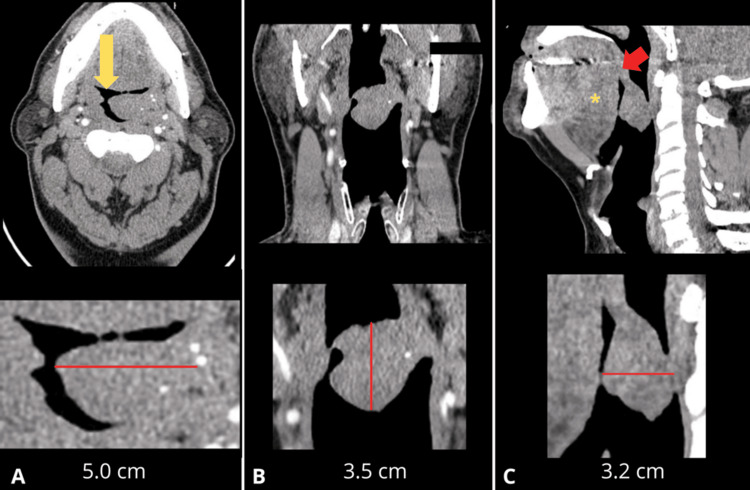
Contrast-enhanced computed tomography showing the left oropharyngeal tumor (A) Sagittal view. (B) Coronal view. (C) Axial view. A well-defined, saccular-appearing mass originates from the anterior wall of the oropharynx (red arrow), measuring 5.0 cm in the axial plane, 3.5 cm in the coronal plane, and 3.2 cm in the sagittal plane. The lesion occupied the left tonsillar fossa and extended medially into the oropharyngeal lumen, resulting in approximately 60%-70% narrowing of the parapharyngeal airway column (yellow arrow). Inferiorly, the mass was in close anatomical relationship with the floor of the tongue (yellow asterisk), without evidence of infiltration. The pharyngeal constrictor muscles maintained their normal appearance without signs of compression or invasion, and the carotid space structures, including the internal carotid artery and internal jugular vein, were preserved with no evidence of displacement or encasement. No pathologically enlarged cervical lymph nodes were identified
